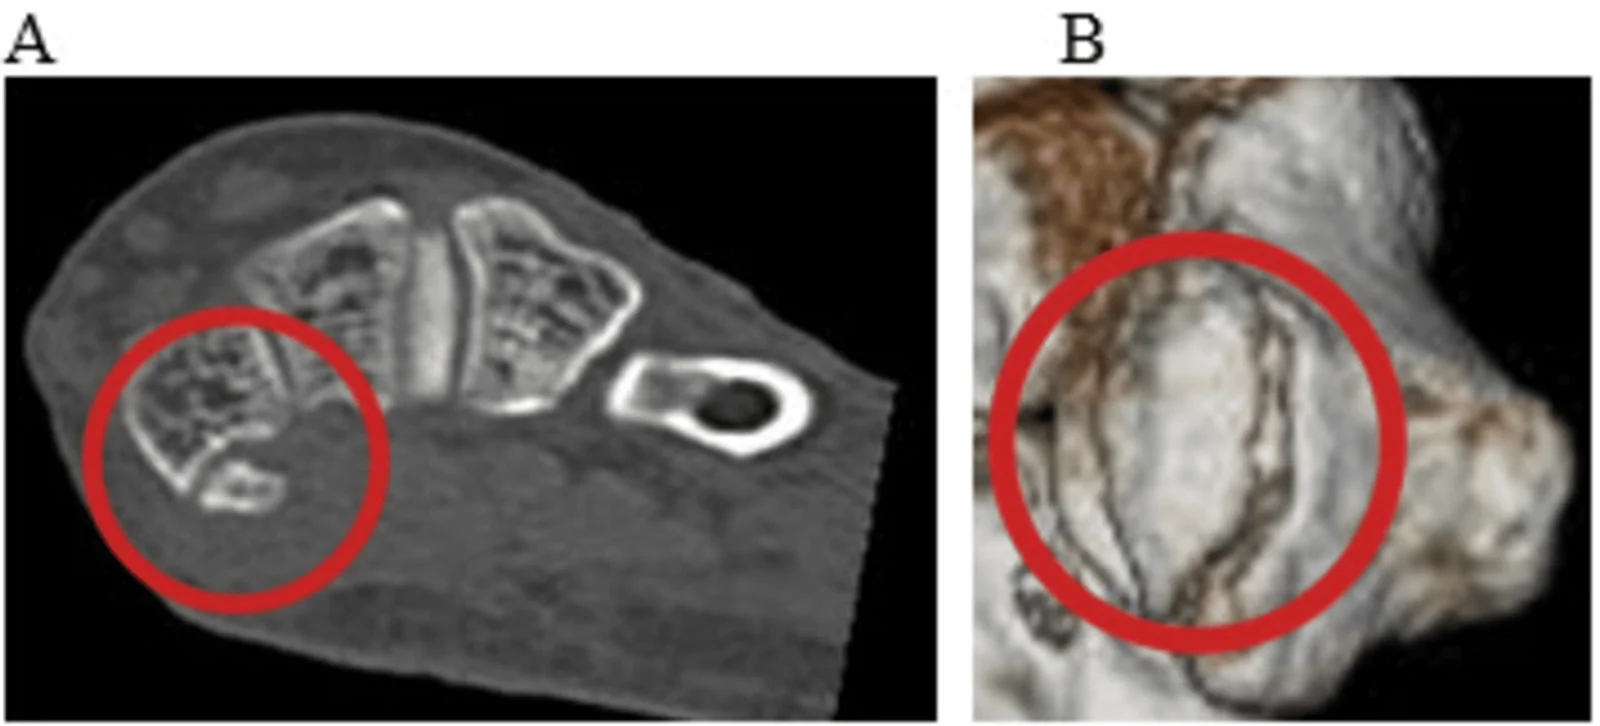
Case 2: Final CT images obtained nine weeks post-injury. Axial (A) and three-dimensional (B) CT images showing similar results to those at four weeks – both fracture exacerbation and callus formation were absent (red circles).
